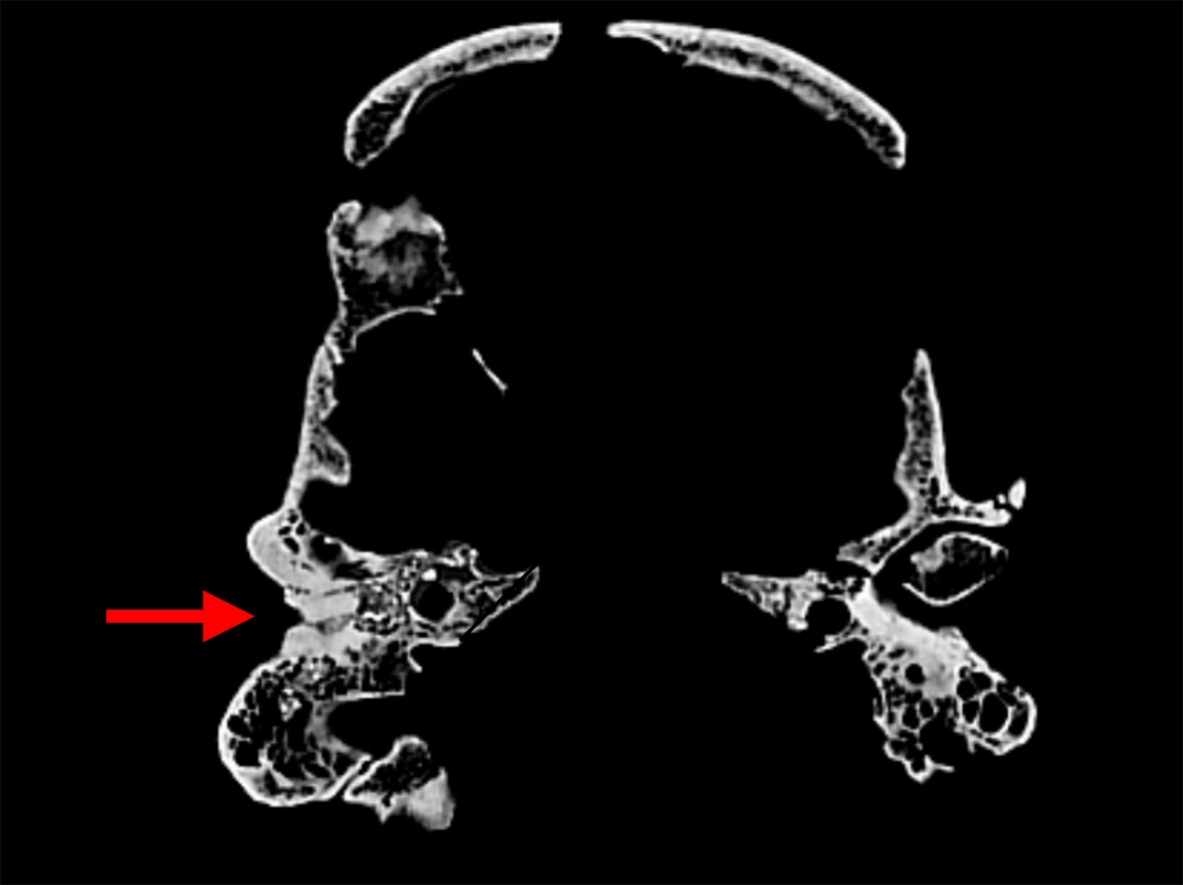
CT-scan aspect of right auricular canal exostosis of skeleton 19.2 with a partial closing of the external ear canal (red arrow / picture P. Charlier)
